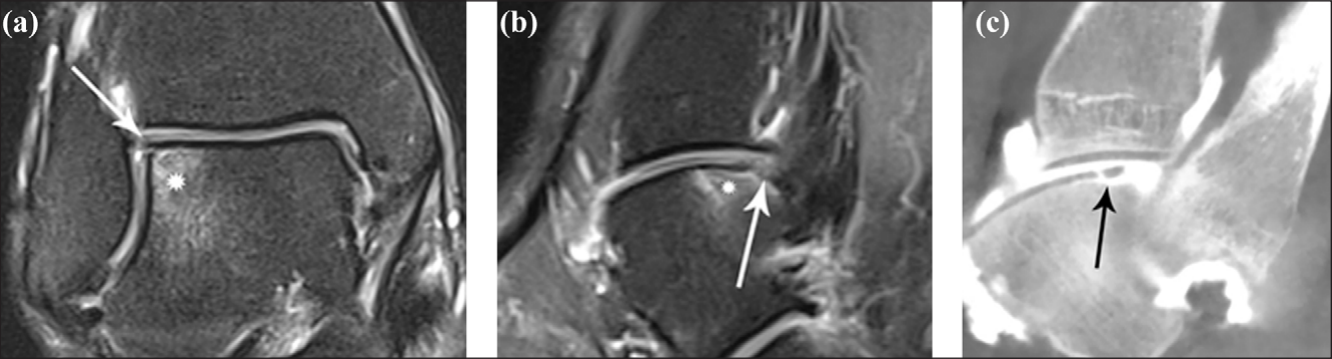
Example of understaging of the cartilage defect of an OCL on MRI compared to CBCT arthrography. Coronal fat suppressed T2-WI (intermediate weighting) showing BME (white asterisk) at the lateral corner of the talar dome (a). There is slight irregular delineation of the cartilage (white arrow). Sagittal fat suppressed T2-WI (intermediate weighting) showing BME (white asterisk) at the lateral corner of the talar dome (b). There is slight irregular delineation of the cartilage (white arrow). On a corresponding sagittal reformatted CBCT-A (c), there is almost complete detachment of the cartilage at the superolateral aspect of the talar dome (black arrow).
